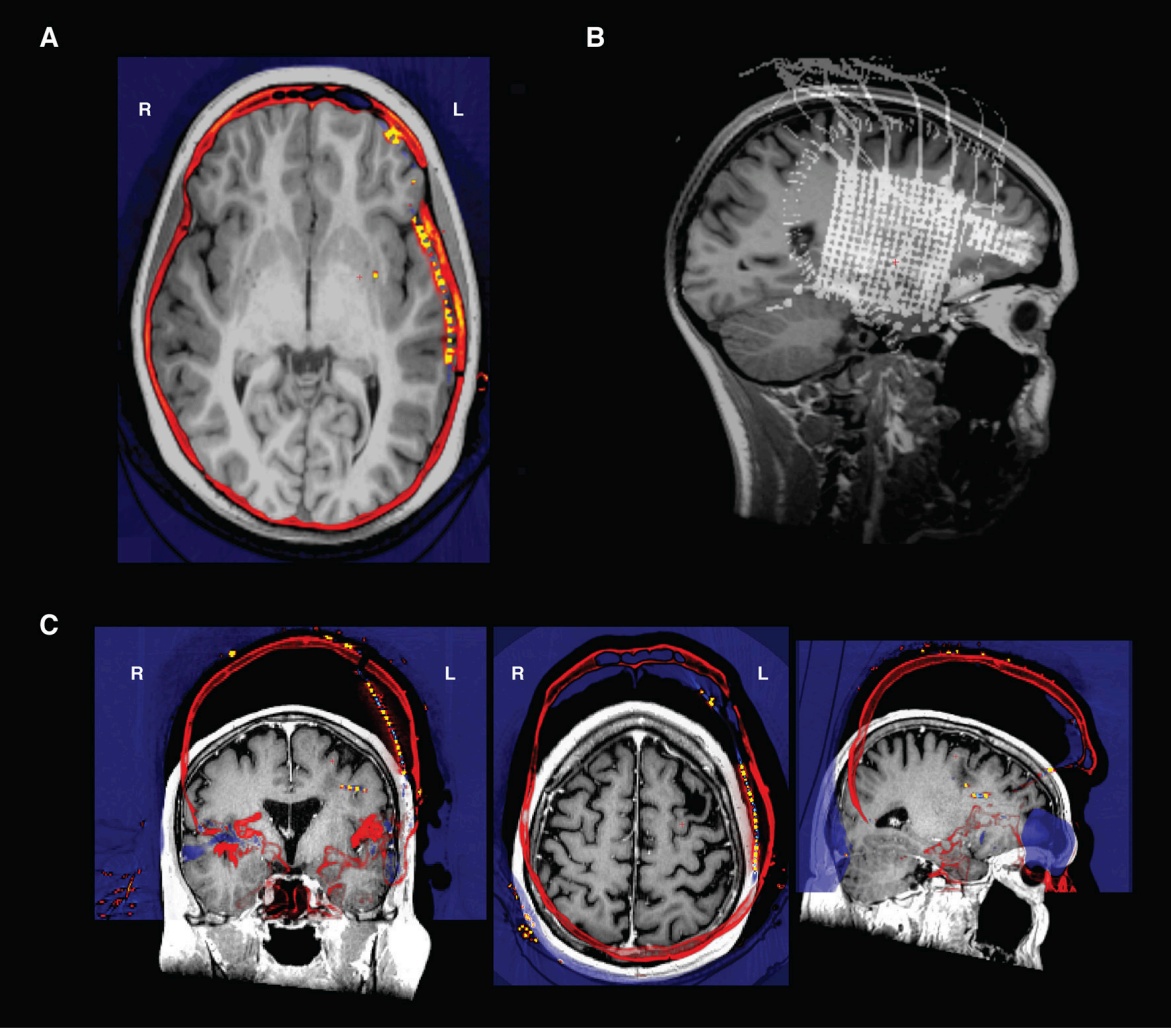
(A) Transverse view in Freeview of a CT and an MRI that have been co-registered- the electrodes are the orange points in the left hemisphere. Note how the skull in both the CT and MRI are aligned after registration. (B) An intensity projection view. (C) CT and T1 scan in original native space before alignment. The CT shown here (in “heat” colormap) was unable to be aligned to the T1 scan (grayscale image) because of poor initial conditions. To align, the CT was translated and rotated to be in rough alignment with the T1 scan, and then reregistered in img_pipe.
